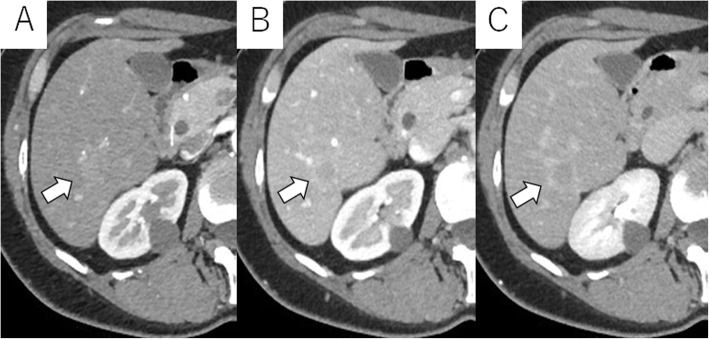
On CT, the nodule is slightly enhanced in the arterial dominant phase (a). The lesion shows perinodular enhancement in the portal and delayed phases (b, c) (white arrow)
